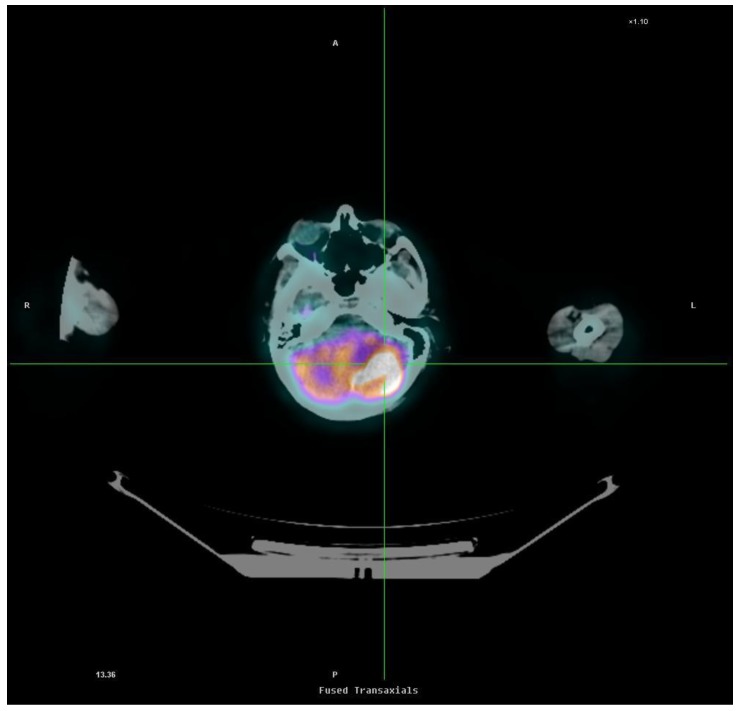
2-Deoxy-[^18^F]fluoro-d-glucose(^18^FDG), positron emission tomography(PET)/computerized tomography (CT) image (transaxial section) of a patient with a metastasis in the left cerebellum deriving from non-small-cell-lung cancer (NSCLC). The metastasis appears as an area of hyperfixation of ^18^FDG in the context of normal cerebellar parenchyma with homogeneous and symmetrical radiopharmaceutical distribution.
